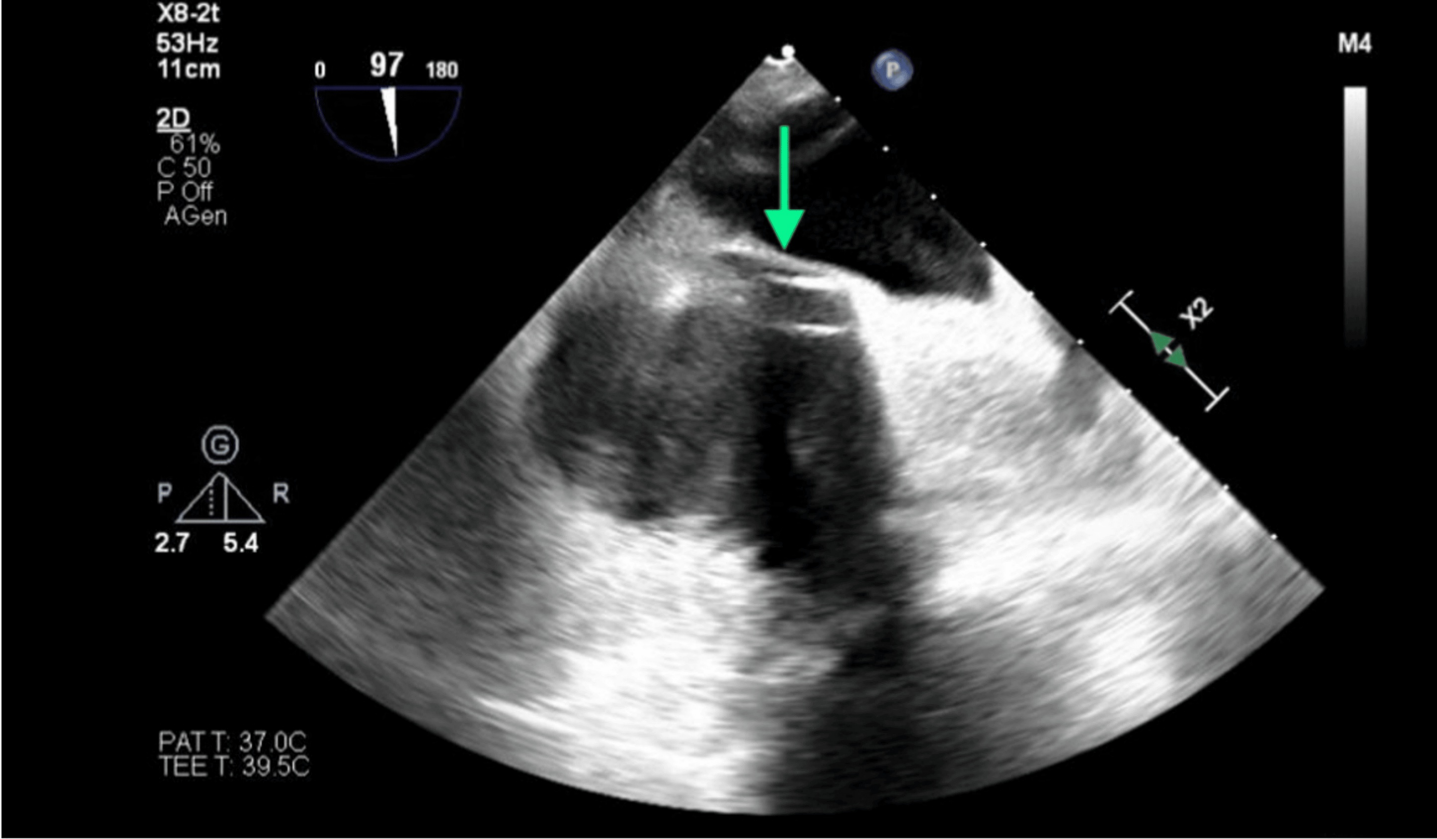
Ultrasound guidance of venous cannulation.
